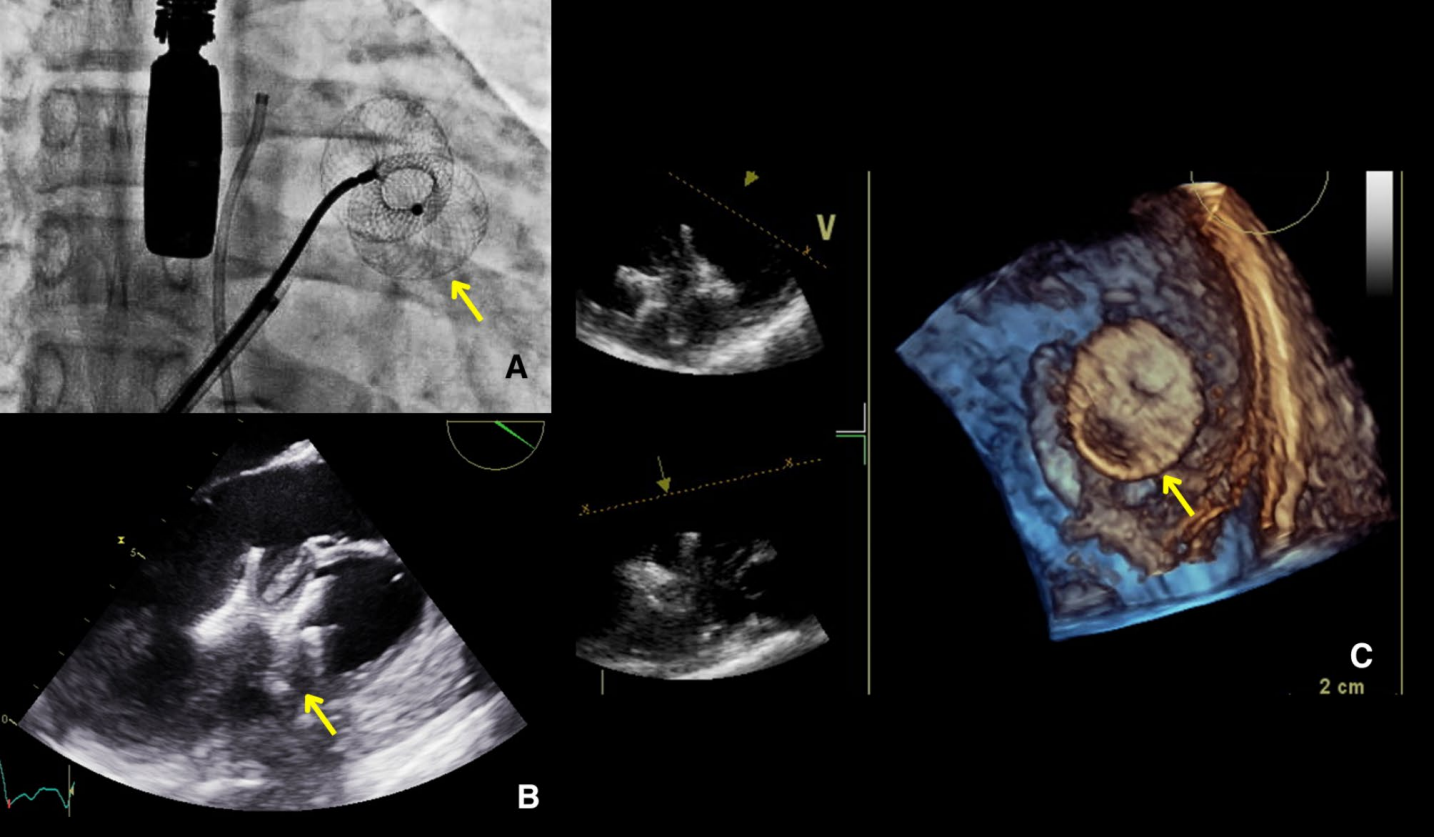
Intraoperative fluoroscopic and transesophageal echocardiogram (TEE) guidance. A. Fluoroscopy showing the deployment of an Amplatzer device (yellow arrow). B. TEE Parasternal long-axis window: Amplatzer device well positioned (yellow arrow). B. TEE 3D reconstruction of the Amplatzer device (yellow arrow) occluding the VSD
